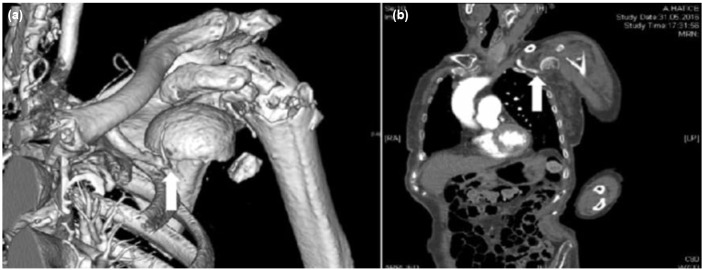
CT angiography of the shoulder (a) 3D Image and (b) Standard Image.
